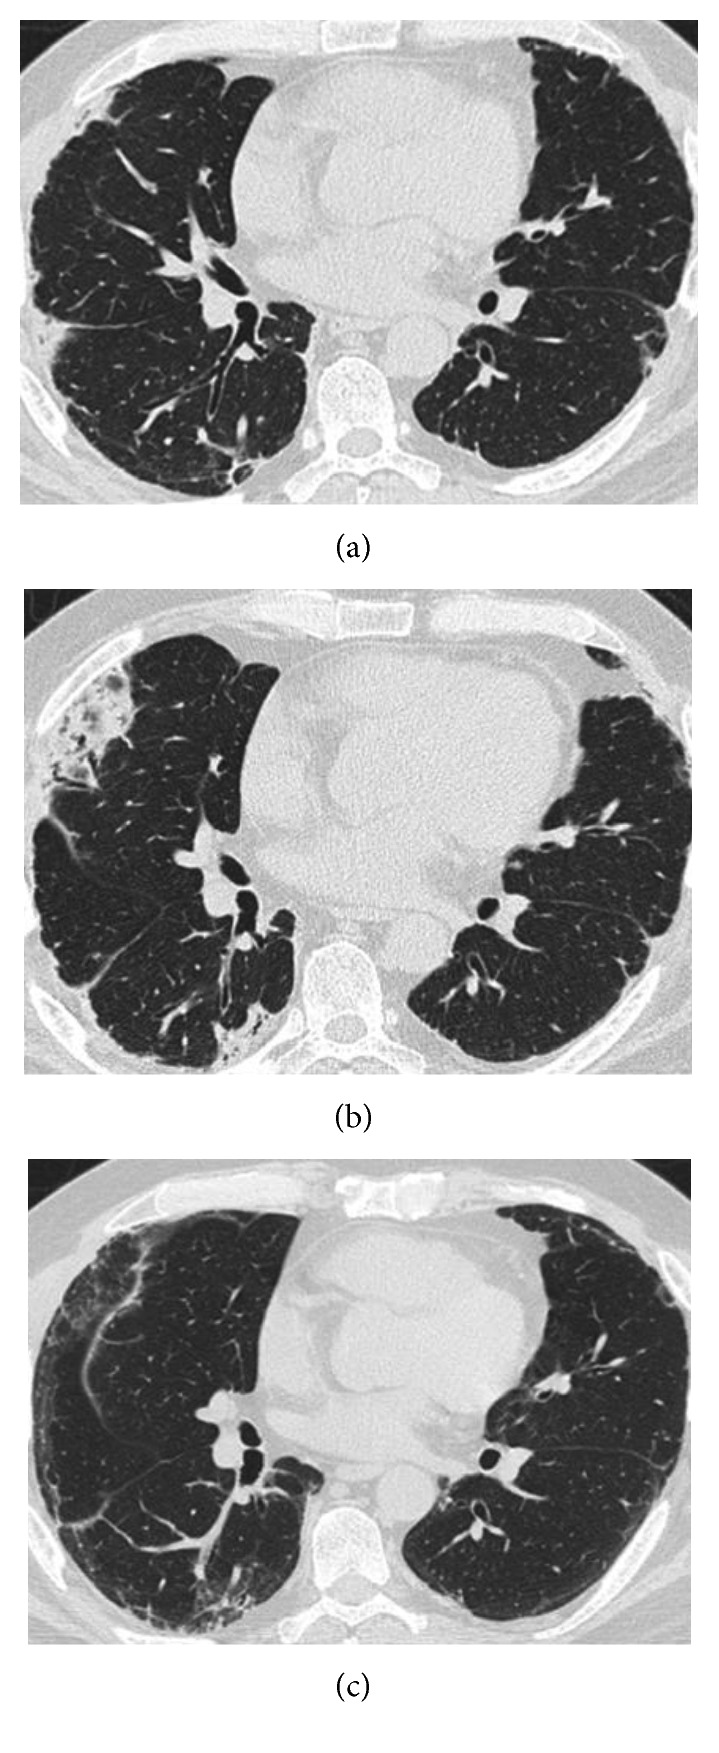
Chest computed tomography findings at admission (a), before the addition of rituximab (RTX) (b), and after RTX administration (c).
